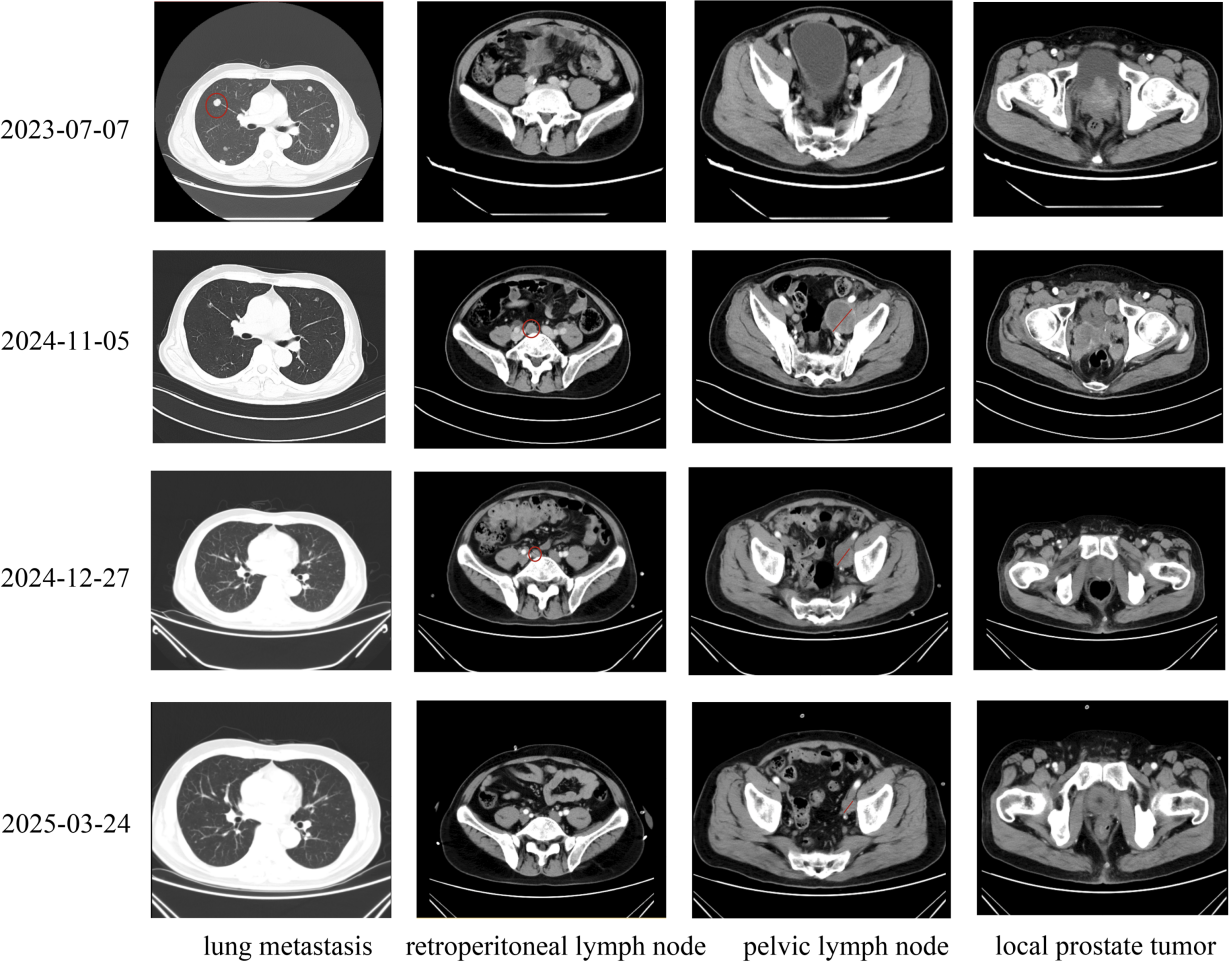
Timeline of Imaging Changes in Prostate Cancer Metastases Across Diagnosis, Treatment, and Follow-Up: On July 6, 2023, enhanced computed tomography (CT) scans of the chest, abdomen, and pelvis revealed multiple metastatic lesions in the lungs, locally advanced prostate tumors protruding into the bladder, small metastatic lymph nodes in the pelvis, and no evidence of retroperitoneal lymph node involvement in a patient diagnosed with prostate cancer. Following laparoscopic radical prostatectomy in September 2024, the patient declined immediate adjuvant chemotherapy. Subsequent enhanced CT scans performed on November 6, 2024, demonstrated rapid tumor progression, with enlarged pelvic lymph nodes, tumor recurrence in the surgical bed, and new metastatic lymph nodes in the retroperitoneum. Notably, pulmonary metastases had largely resolved following prior treatment. After initiating two cycles of cisplatin and etoposide chemotherapy combined with immunotherapy, enhanced CT scans on December 27, 2024, showed a marked reduction in the size of pelvic and retroperitoneal lymph nodes and tumor tissue in the surgical bed, indicating a significant therapeutic response. Following completion of six cycles of combined chemotherapy and immunotherapy, enhanced CT scans on March 24, 2025, revealed near-complete resolution of pulmonary metastases, retroperitoneal lymph nodes, and tumors in the surgical bed, with further reduction in left pelvic lymph nodes, confirming a robust and sustained treatment response.
